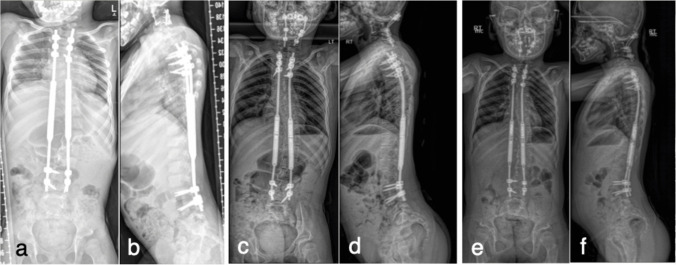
a Full spine radiographs at age 5 years 7 months showing moderate S-shaped thoracolumbar scoliosis with initial MAGEC rod placement from T2–T4 to L3–L4, status post C2–C4 fusion. b Age 8 years 10 months demonstrating maintained alignment with exchanged growing rods, progression of basilar invagination, and new occipital-to-C5 fusion construct. c Age 9 years 10 months revealing stable constructs with appropriate interval lengthening and no hardware complications
